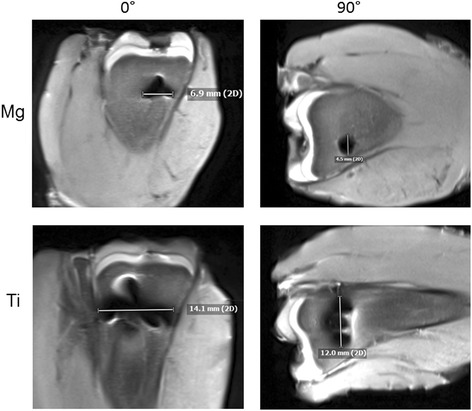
Artifact appearance in 3 T MRI PDw TSE (FS) in two positions of the screw (0°/90°)
